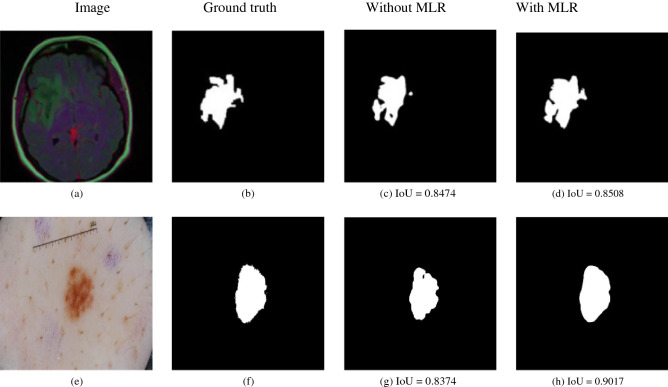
Two visual examples from the MRI^15^
